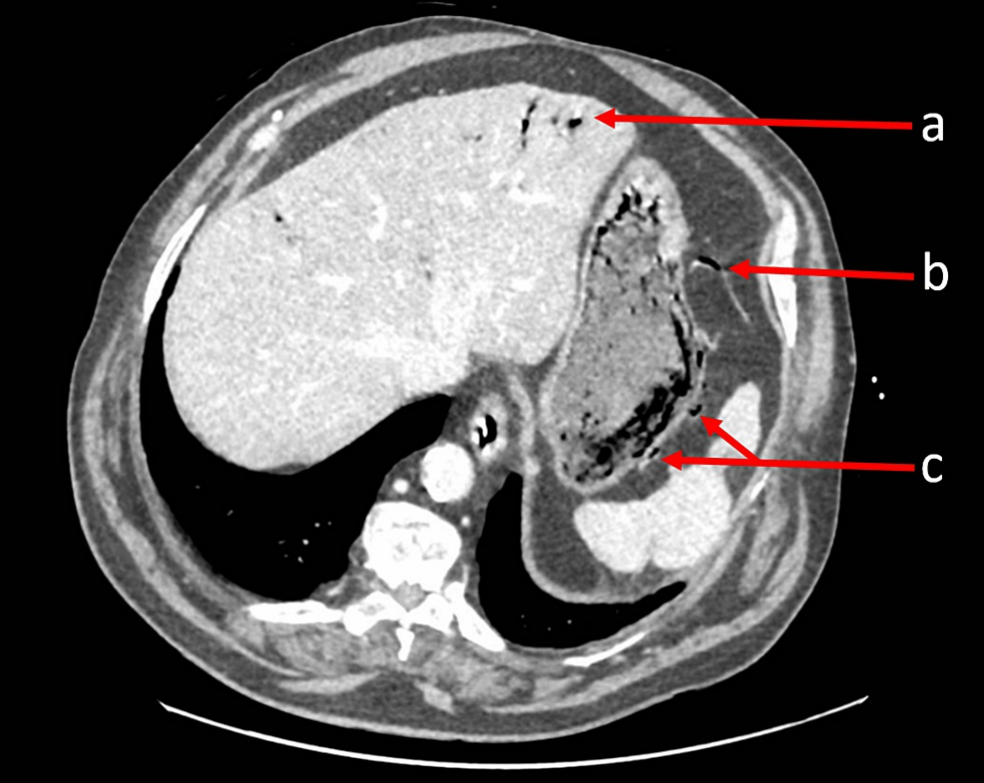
Axial portal-venous phase CT abdomen and pelvis (a) Hepatic portal venous gas. (b) Mesenteric venous gas. (c) Gastric intramural gas locules.
